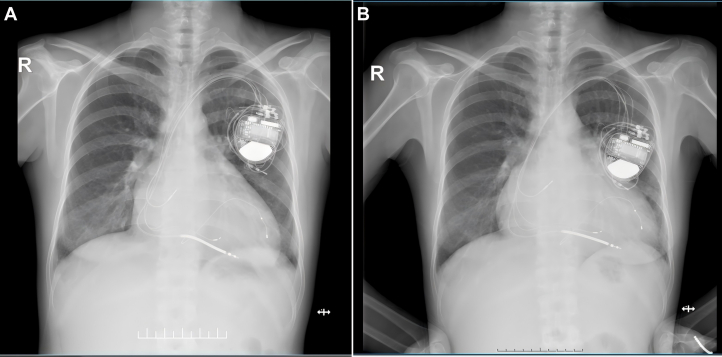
Serial Chest X-ray Imaging (A) Chest x-ray post-cardiac resynchronization therapy procedure. (B) Chest x-ray at 1-year follow-up after CRT implantation.
